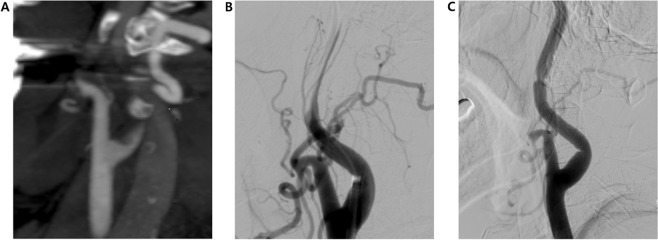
Example of a cervical ICA pseudo-occlusion on CTA with a non-corresponding cause of apparent occlusion found on DSA. (A) Pseudo-occlusion of the left cervical ICA in a 69-year-old woman. CTA shows a sharp, flame-shaped leading contrast edge at the level of the cervical ICA, with T-occlusion present. (B) DSA of this patient shows contrast moving slowly upward and finally a patent ICA (C) after mechanical thrombectomy of the distal ICA occlusion.
